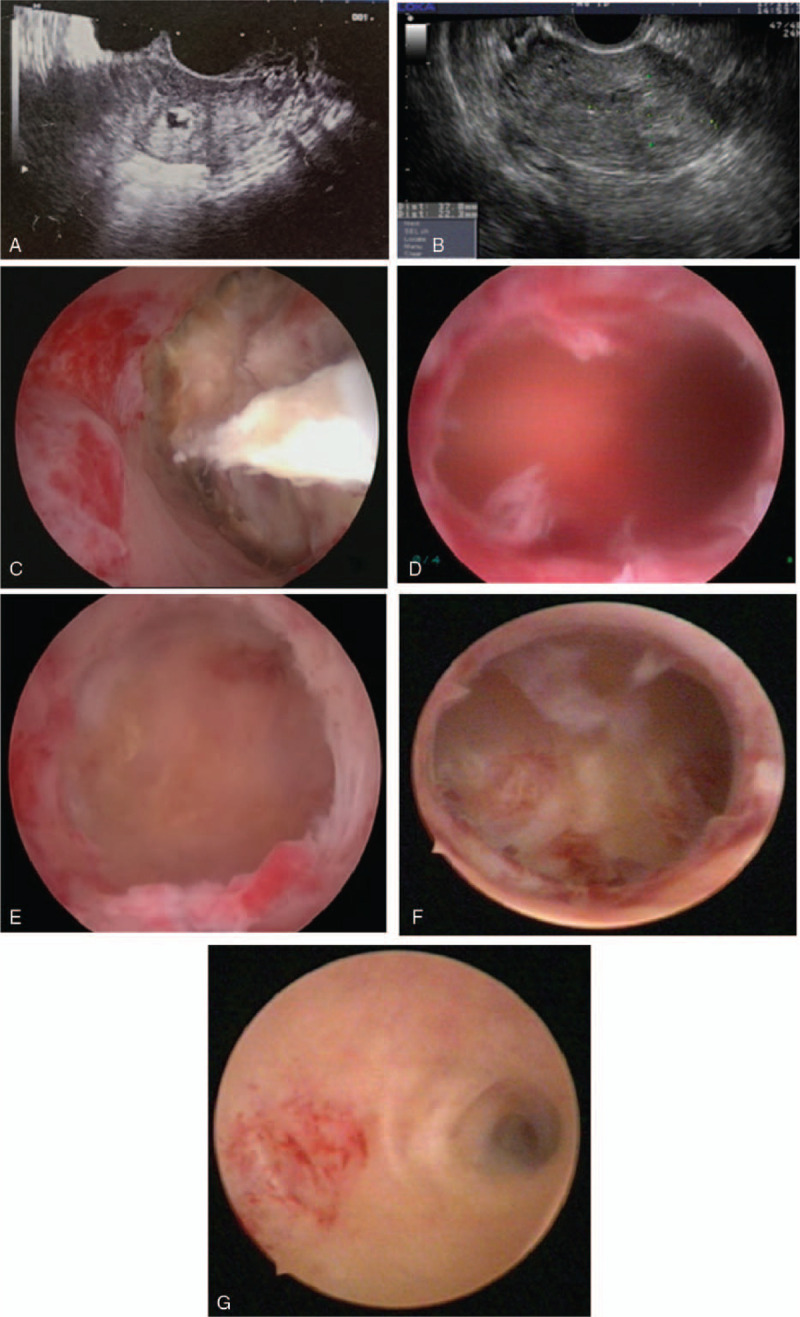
(A) The local ultrasound image of gestational sac, (B) ultrasound image of placental remnants, (C) hysteroscopic view of placental remnants in the left uterine horn, (D) hysteroscopic view of uterine cavity after complete resection of placental remnants, (E) hysteroscopic view of left uterine horn after complete resection of placental remnants, (F) second-look hysteroscopic view of uterine cavity, and (G) second-look hysteroscopic view of left uterine horn.
